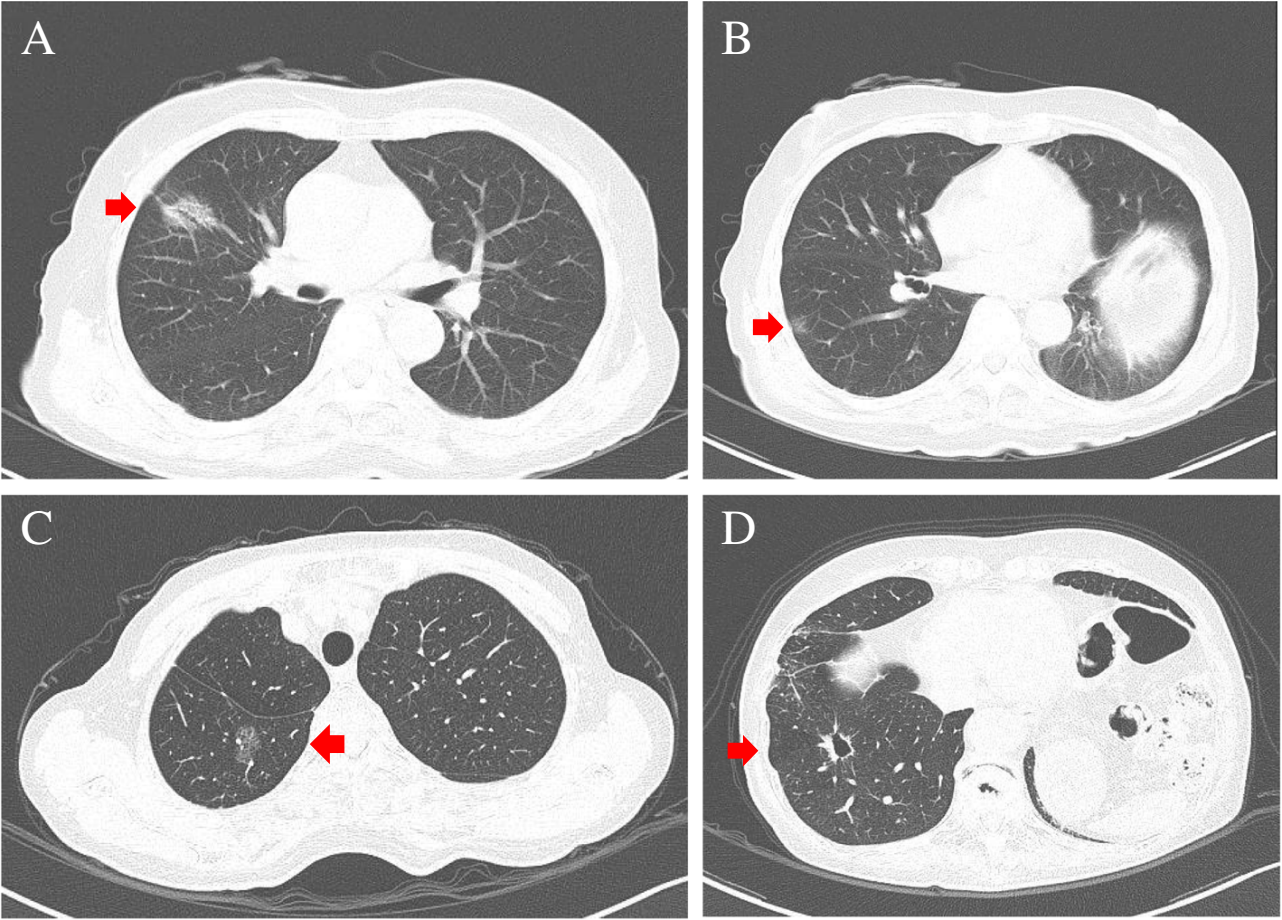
Chest CT showing multiple lung tumors. a Right S^2^ tumor. The size was 28 mm. b Right S^6^b peripheral tumor. The size was 10 mm. c Right S^6^a pure ground-glass nodule. The size was 27 mm. d Right S^9^ solid tumor near a bulla. The size was 21 mm
